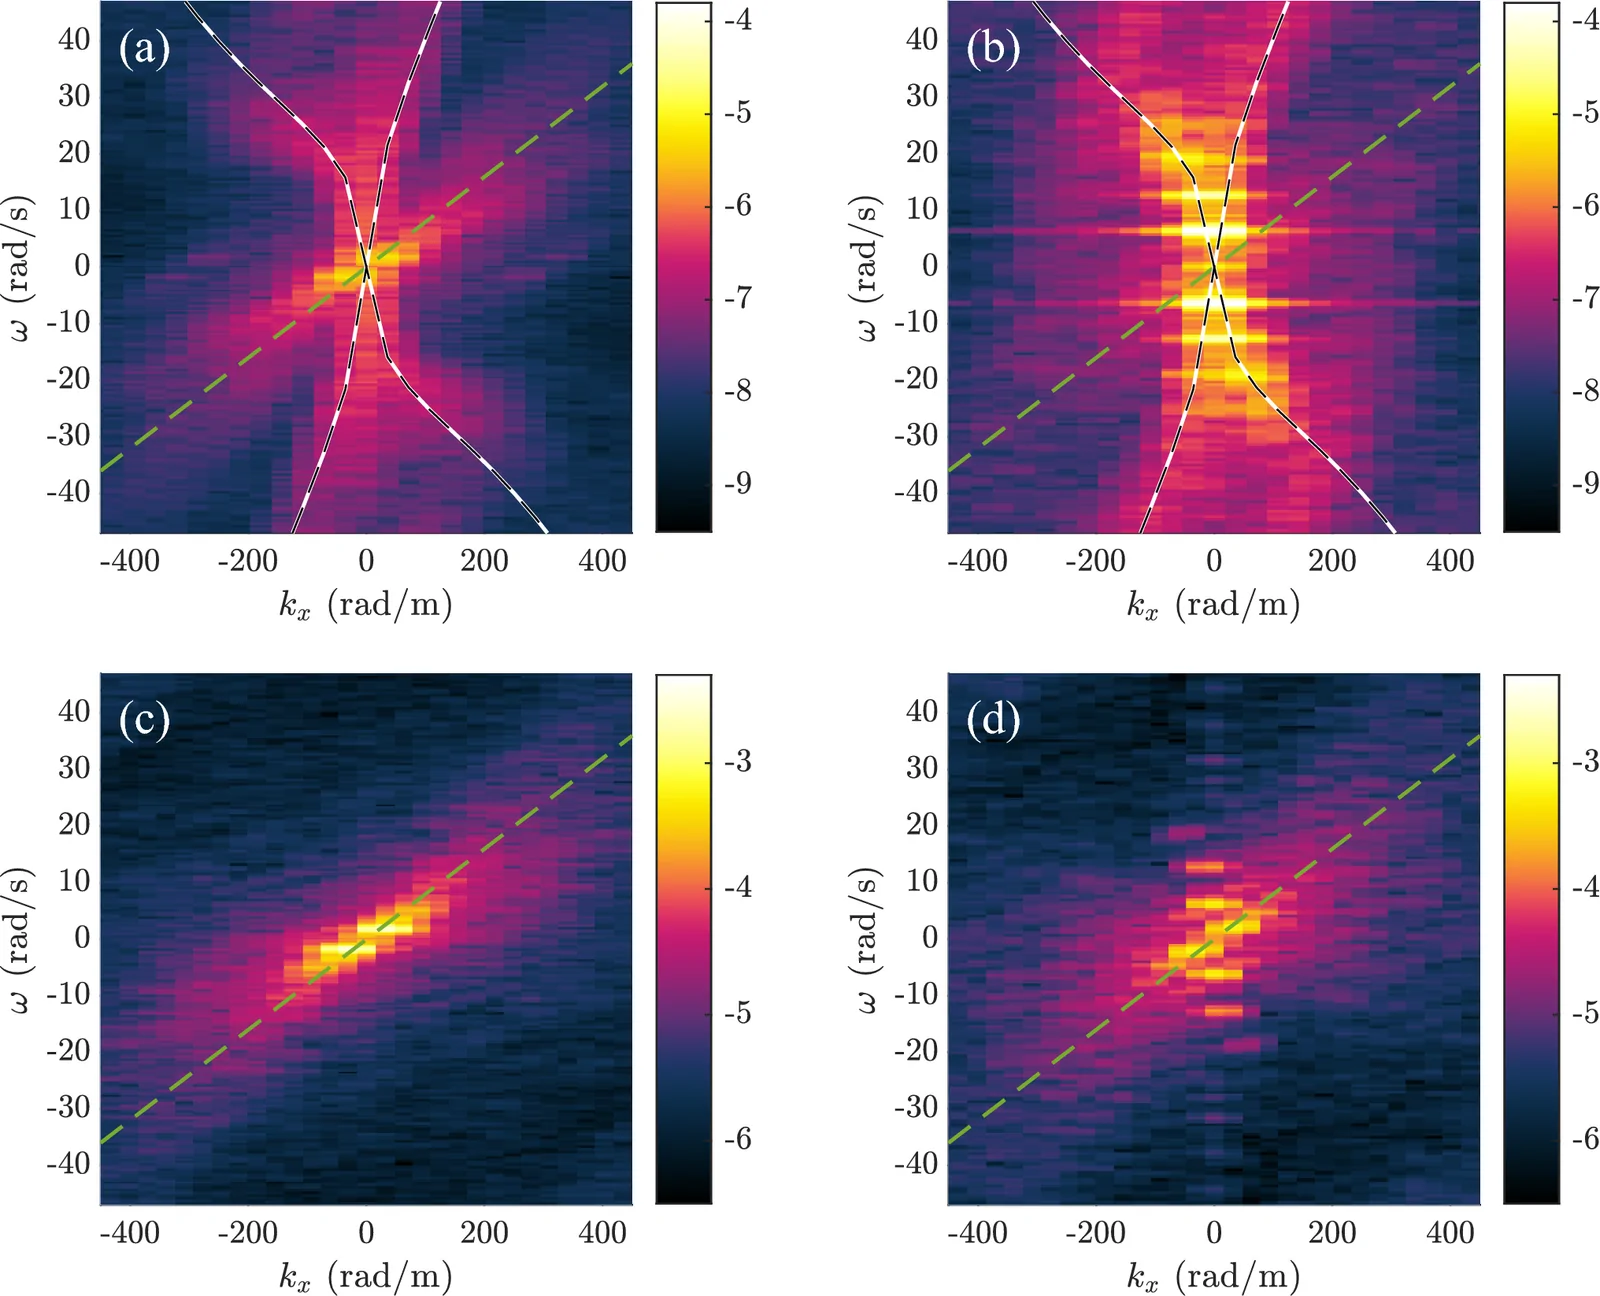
Wavenumber–frequency spectra $$\log _{10} E(k,\omega )$$ of the surface elevation (top row) and the transverse velocity component (bottom row). The left column shows pure vortex shedding from a cylinder (a,c), while the right column (b,d) shows vortex shedding coupled with surface waves. The black and white dashed curves show the Doppler-shifted linear dispersion relation, and the dashed green line indicates the convection line $$\omega =U_\textrm{avg} k_x$$, where $$U_\textrm{avg} = 0.08$$ m/s
